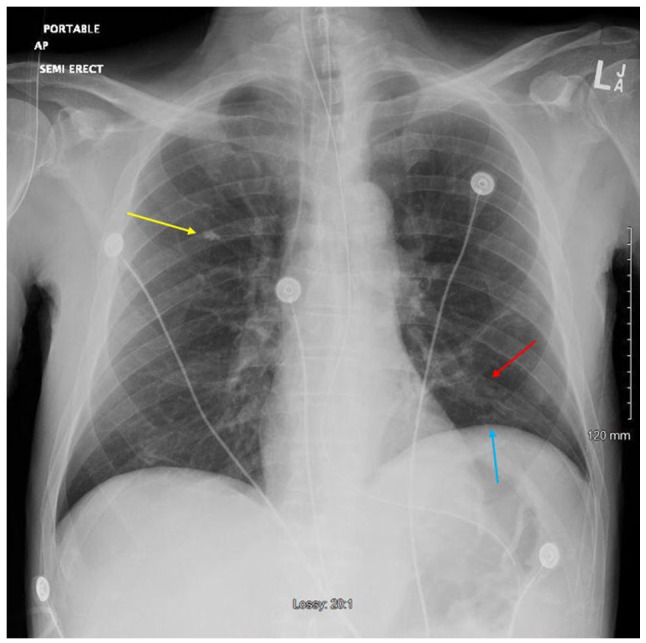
Chest x-ray taken on admission showing left basilar atelectasis (red arrow), left hemi diaphragm elevation (blue arrow), and a right upper lobe irregular calcification (yellow arrow).
